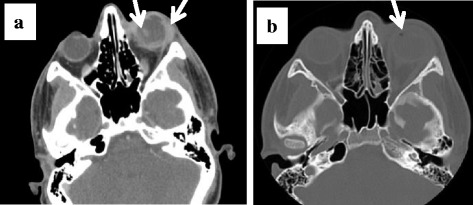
a Orbital computed tomography showing proptosis, posterior dislocation of the lens, thickening of the periorbital soft tissue and the posterior wall of the globe. b Gas bubbles in the left eye
